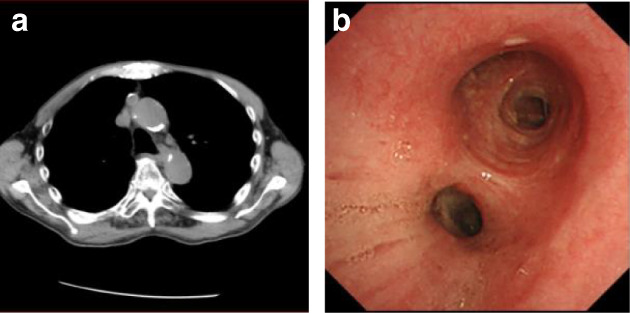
(a) Computed tomography (CT) and (b) bronchoscopy after pembrolizumab treatment. The tumor had completely disappeared at the end of the fifth cycle.
